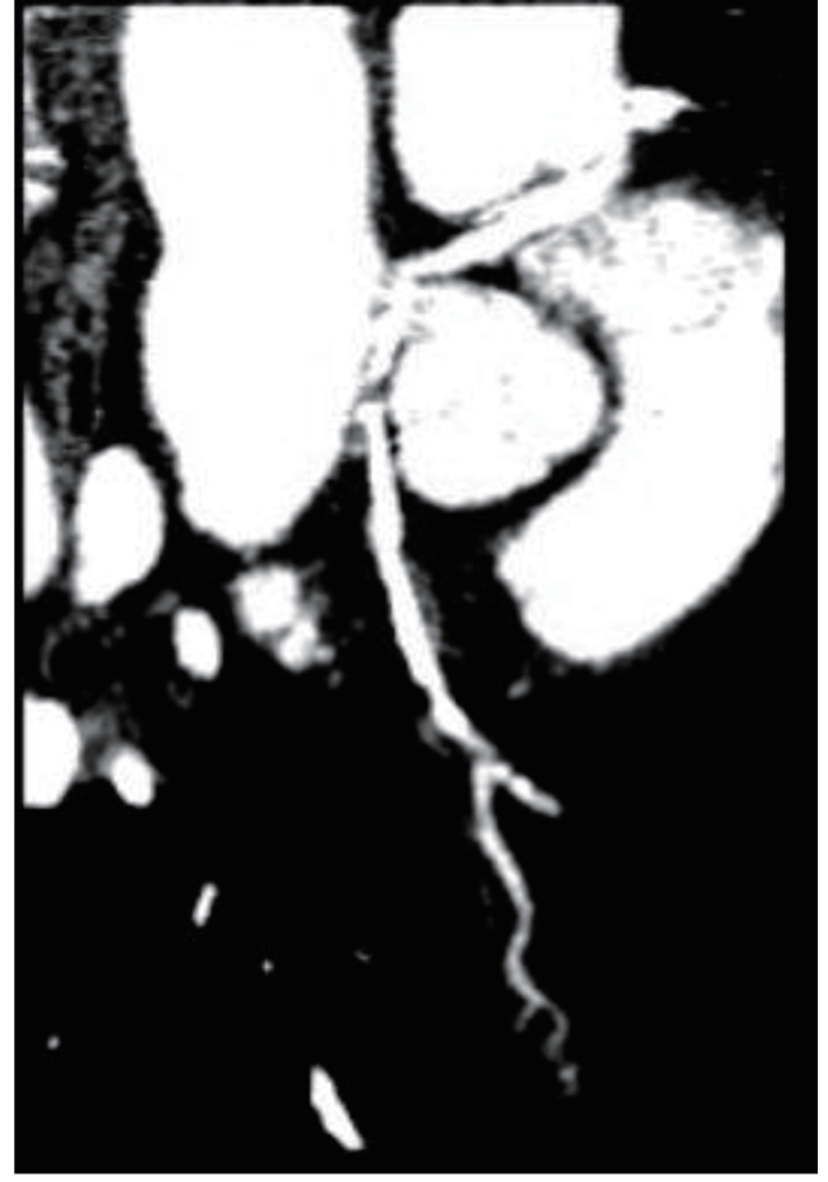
Coronary CT angiogram highlighting the origin of two vessels, the RCA and LCA, from a single coronary cusp.
